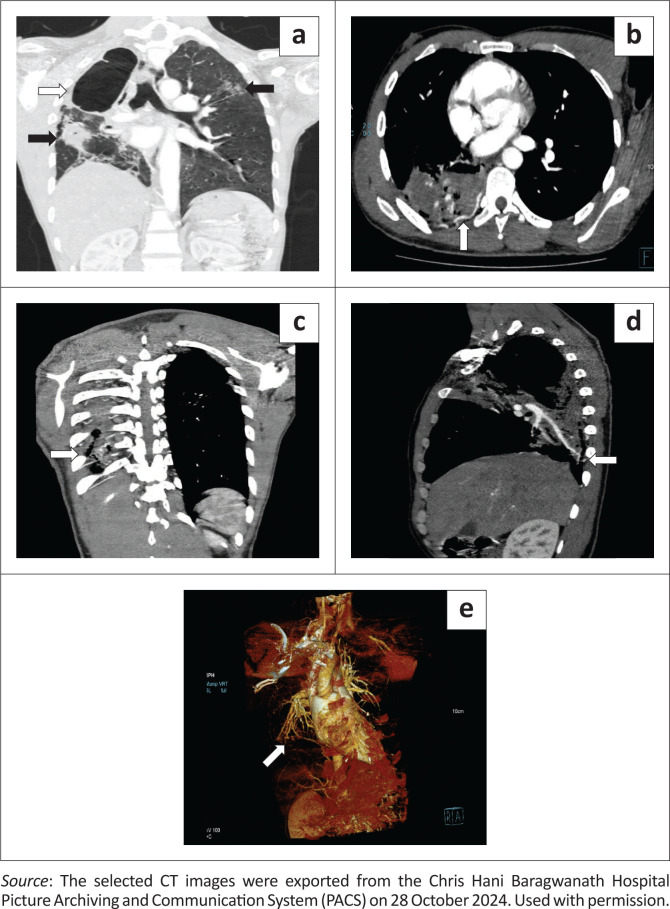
(a) Coronal reconstruction of the contrast-enhanced CT of the chest (lung window) demonstrating the right upper lobe fibrocavitary changes (white arrow) with ipsilateral lung volume loss, tracheal deviation, and opacities in the left upper, and right mid-lung zones with right lower lobe consolidation (black arrows). (b) Axial contrast-enhanced maximum intensity projection (MIP) CT of the chest demonstrating a hypertrophied and tortuous right 9th intercostal artery (white arrow). (c) Coronal contrast-enhanced MIP CT of the chest. (d) Sagittal contrast-enhanced MIP CT of the chest. (e) 3D coronal CT angiogram of the thorax further demonstrates dilatation and tortuosity of the 9th intercostal artery (white arrow).
